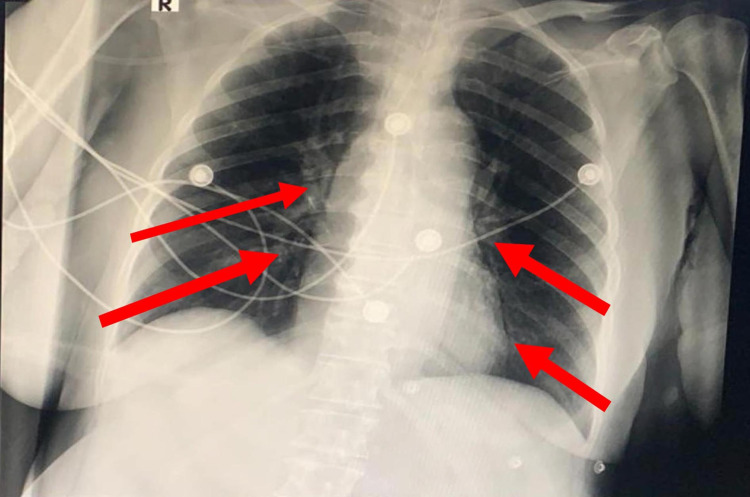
Chest x-ray on the first day of admission Ground glass opacities (red arrows) are seen involving the central and peripheral zones bilaterally
